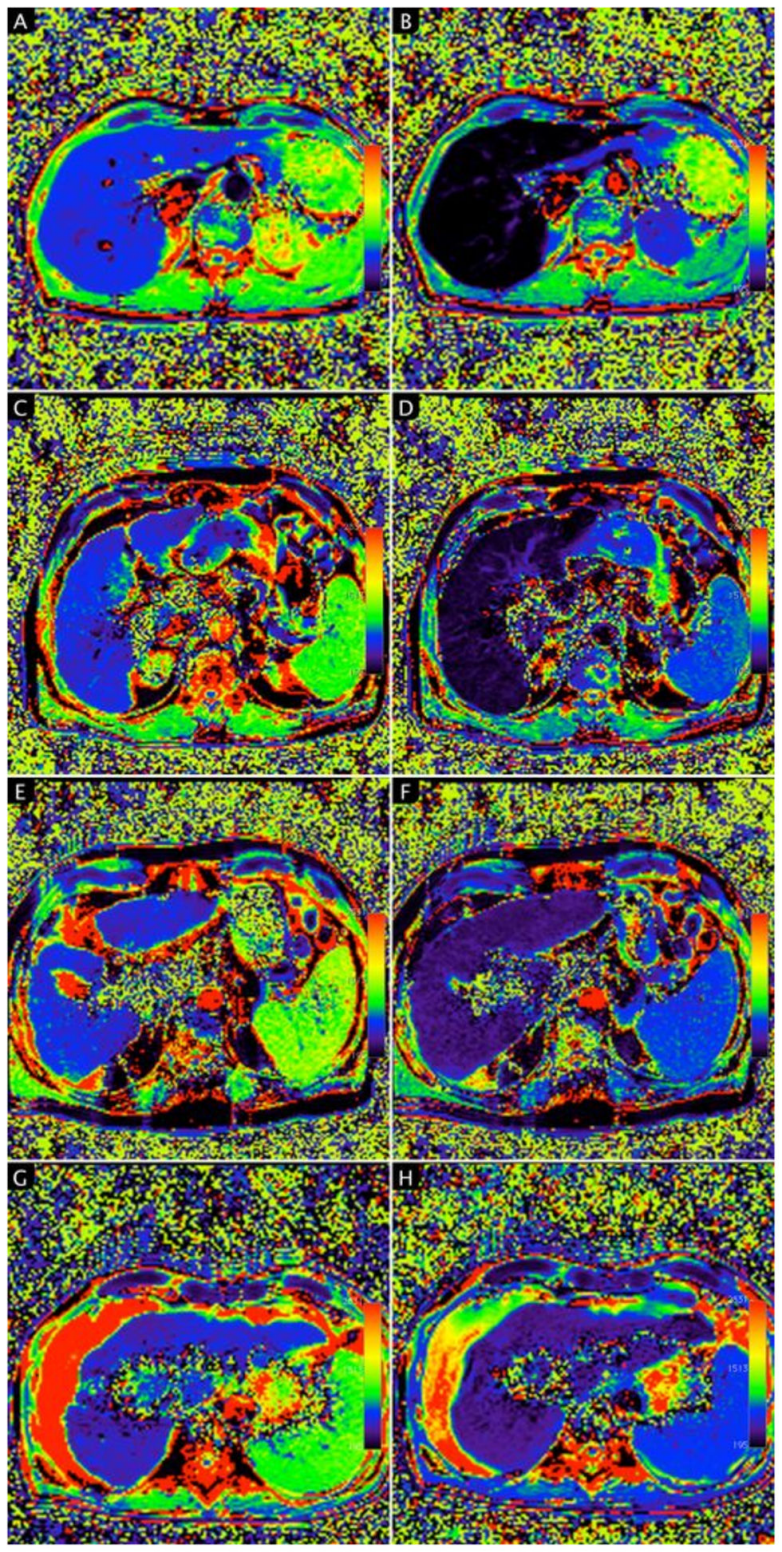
T1 relaxation times of the liver on T1 mapping color-coded images. A - H, T1 mapping images calculated from 2 TurboFlash sequences (TI = 400 ms and 1000 ms) were obtained before (A, C, E, G) and 20 min after Gd-EOB-DTPA administration (B, D, F, H) in a patient with normal liver function (A, B) and patients with liver cirrhosis Child-Pugh A (C, D), Child-Pugh B (E, F), and Child-Pugh C (G, H). The mean T1 relaxation times of liver parenchyma were as follows: 755.6 ms (A), 211.9 ms (B), 717.1 ms (C), 316.0 ms (D), 760.5 ms (E), 434.0 ms (F), 705.7 ms (G) and 482.4 ms (H). The reduction rate of T1 relaxation times in patients with normal liver function and patients with liver cirrhosis Child-Pugh A, Child-Pugh B, and Child-Pugh C were 72%, 56%, 43%, and 32%, respectively. In patients with normal liver function, T1 relaxation times on T1 mapping color-coded images of the liver on post-contrast MRI (B) showed a markedly darker color distribution of the liver parenchyma than that on the pre-contrast mapping image (A), indicating a strong Gd-EOB-DTPA-induced shortening of T1 relaxation time 20 min after contrast medium administration. With increased progression of liver cirrhosis, the color distribution in the liver parenchyma could be visually confirmed to show less change on post-contrast T1- mapping images.
